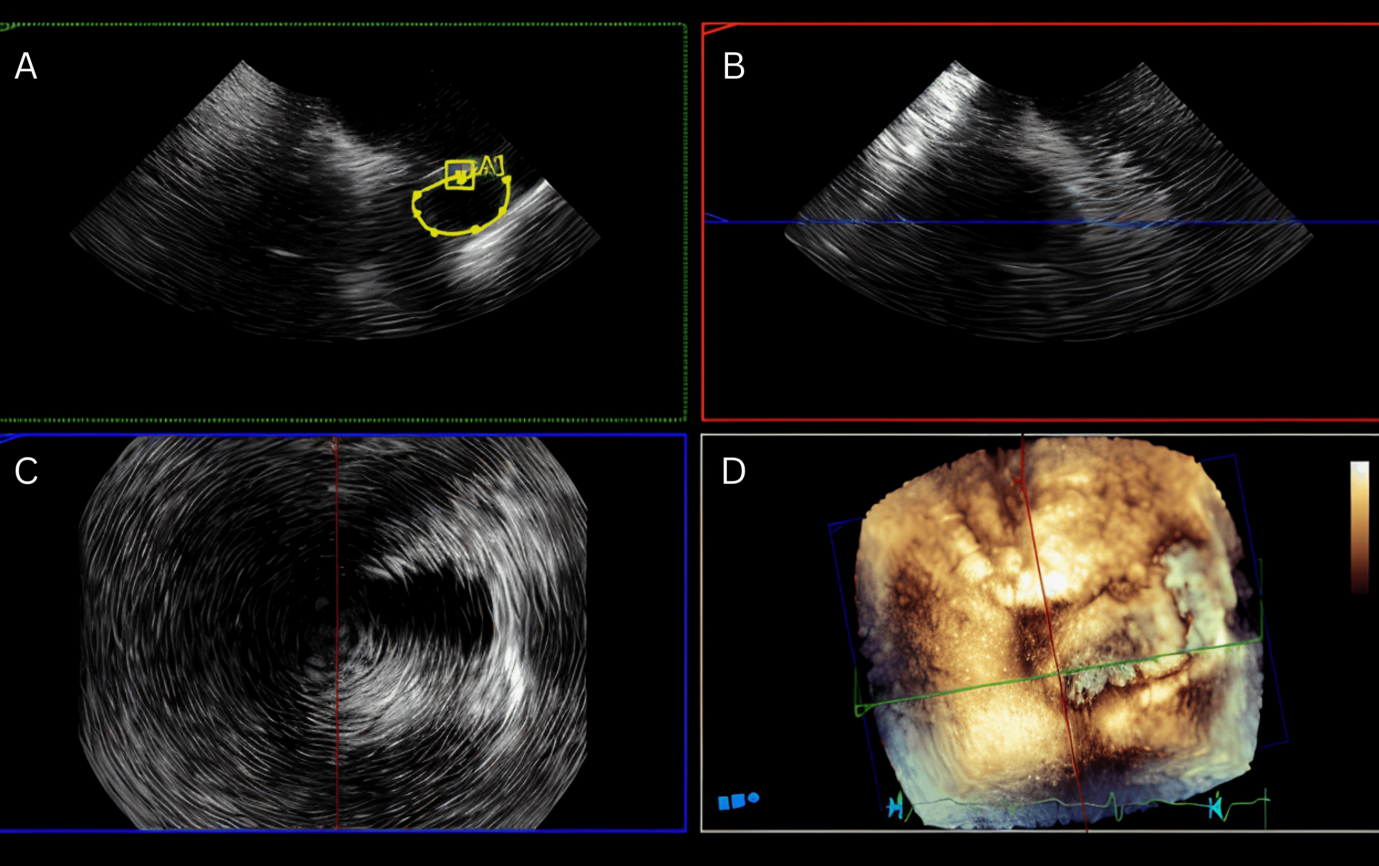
Secundum ASD-TEE views A: A plane of MultiVue on the Philips EPIQ CVx showing a Secundum ASD measuring 2.6cm^2^ (yellow ovoid), B and C: A plane of MultiVue on the Philips EPIQ CVx, D: MultiVue image alignment with 3D rendering visualization of an ASD ASD: atrial septal defect; TEE: transesophageal echocardiography
